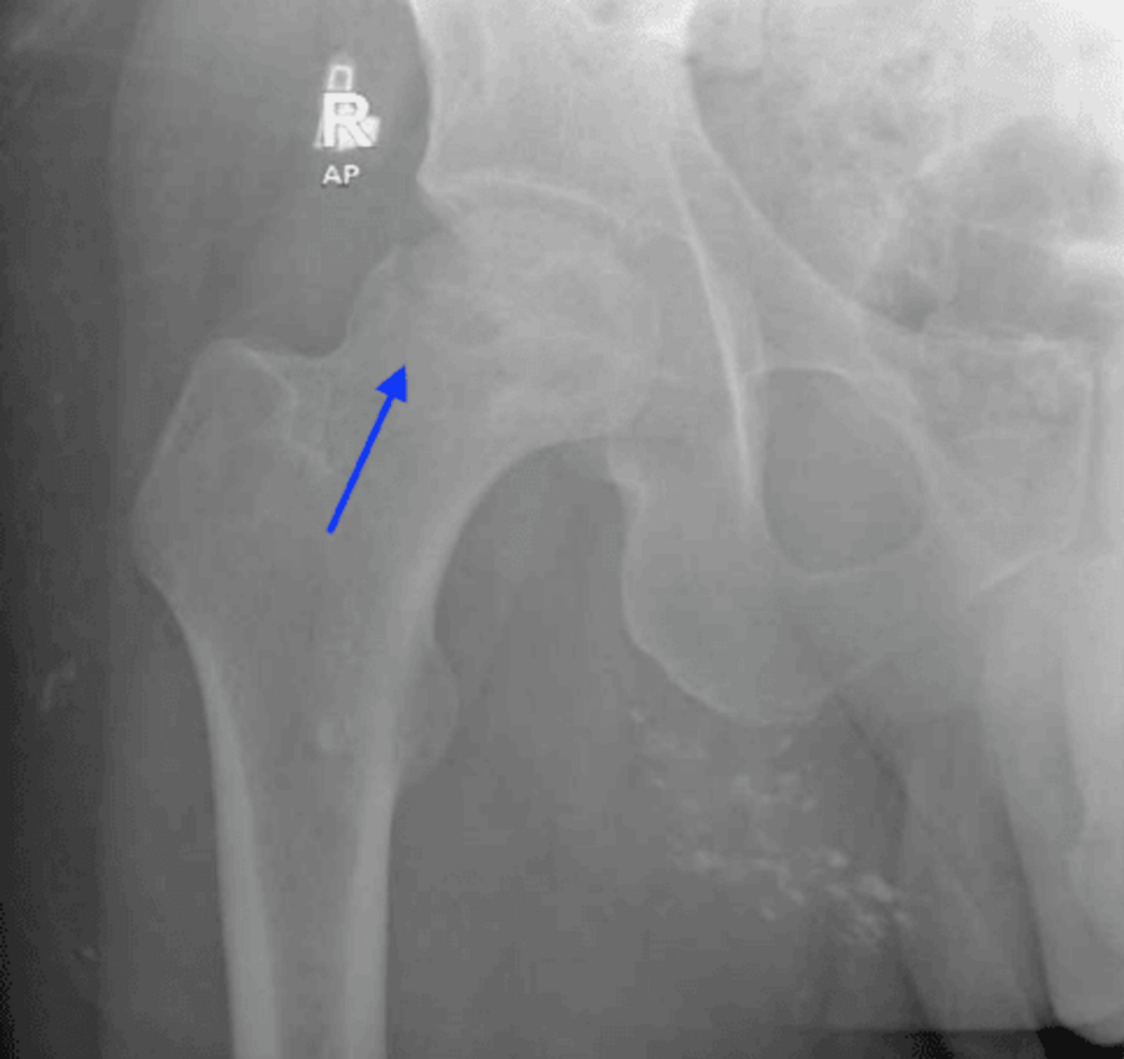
X-ray of the right hip showing focal cortical irregularities in the femoral head and neck junction
